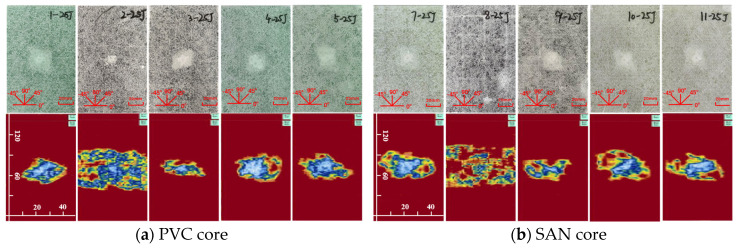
Physical picture of 25 J impact damage and ultrasonic C-scan image.
